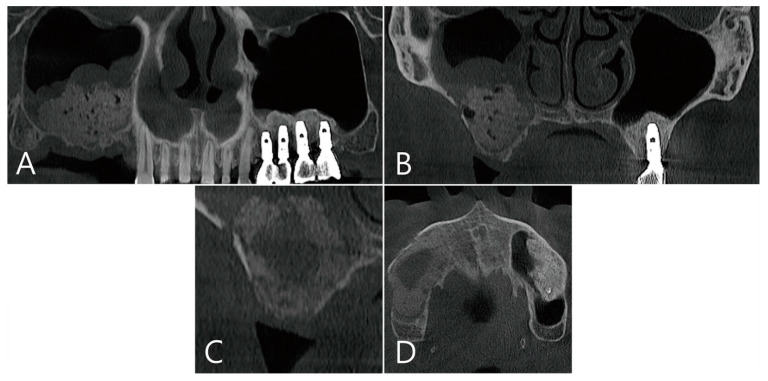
Case 3. CBCT images taken immediately after maxillary sinus bone graft: (A) On the panoramic image of CBCT, a thickened mucosa on the sinus floor was observed above the bone graft; (B) Coronal image of the CBCT. (C,D) Images of the CBCT taken 6 months after surgery: (C) in the coronal image of the CBCT, large grafting voids with a cystic appearance were observed; (D) a well-defined radiolucent appearance was observed on an axial image of the CBCT.
